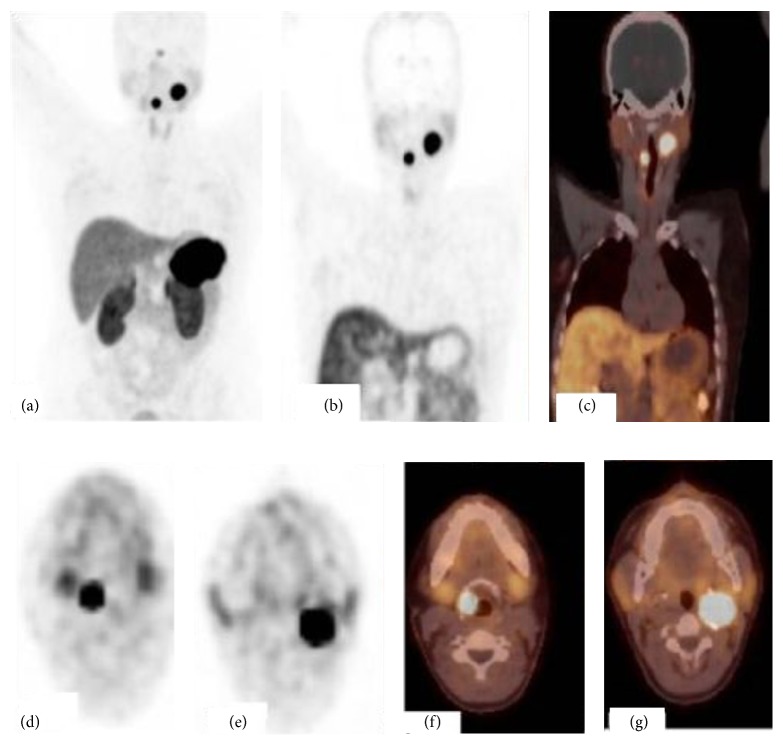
68Ga-DOTANOC PET/CT; maximum intensity projection (MIP) image (a), coronal PET and PET/CT fusion images (b, c), and axial PET and PET/CT fusion images (d, e, f, and g) show an intense uptake by the right laryngeal paraganglioma and left carotid body paraganglioma.
